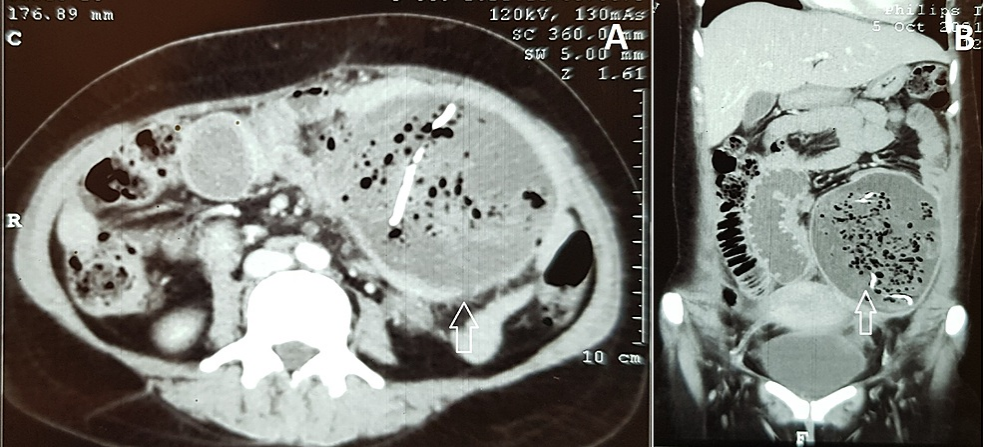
Contrast enhanced computed tomography of the abdomen and pelvis in axial (A) and coronal (B) section showing the large heterogenous lesion in the left iliac fossa containing air foci and linear radio-opaque structures (arrows) suggestive of gossypiboma.
